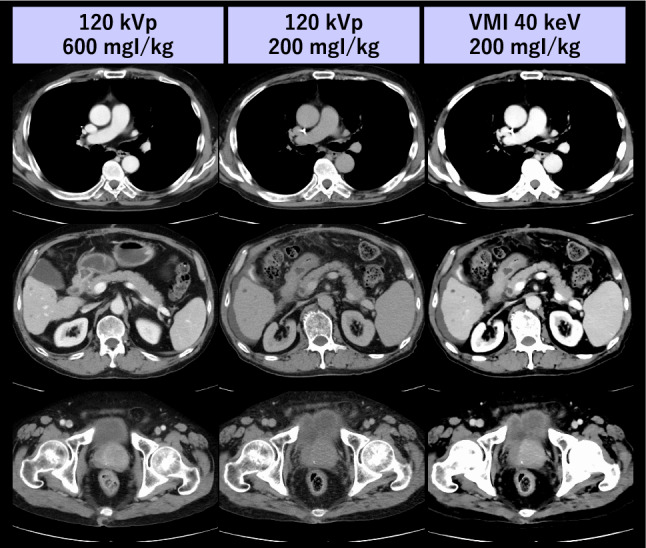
A 72-year-old man with esophageal cancer underwent scanning with both a standard 120 kVp protocol (iodine dose, 37 g; SSDE, 19.2 mGy) and a low ICM dose CT protocol (iodine dose, 12.6 g; SSDE, 16.3 mGy) on single-energy and dual-layer detector CT scanners, respectively, with a 12-month interval. Compared with the 120 kVp images, an equivalent or higher iodine contrast was attained at VMI 40 keV, despite a 66% reduction in iodine dose
